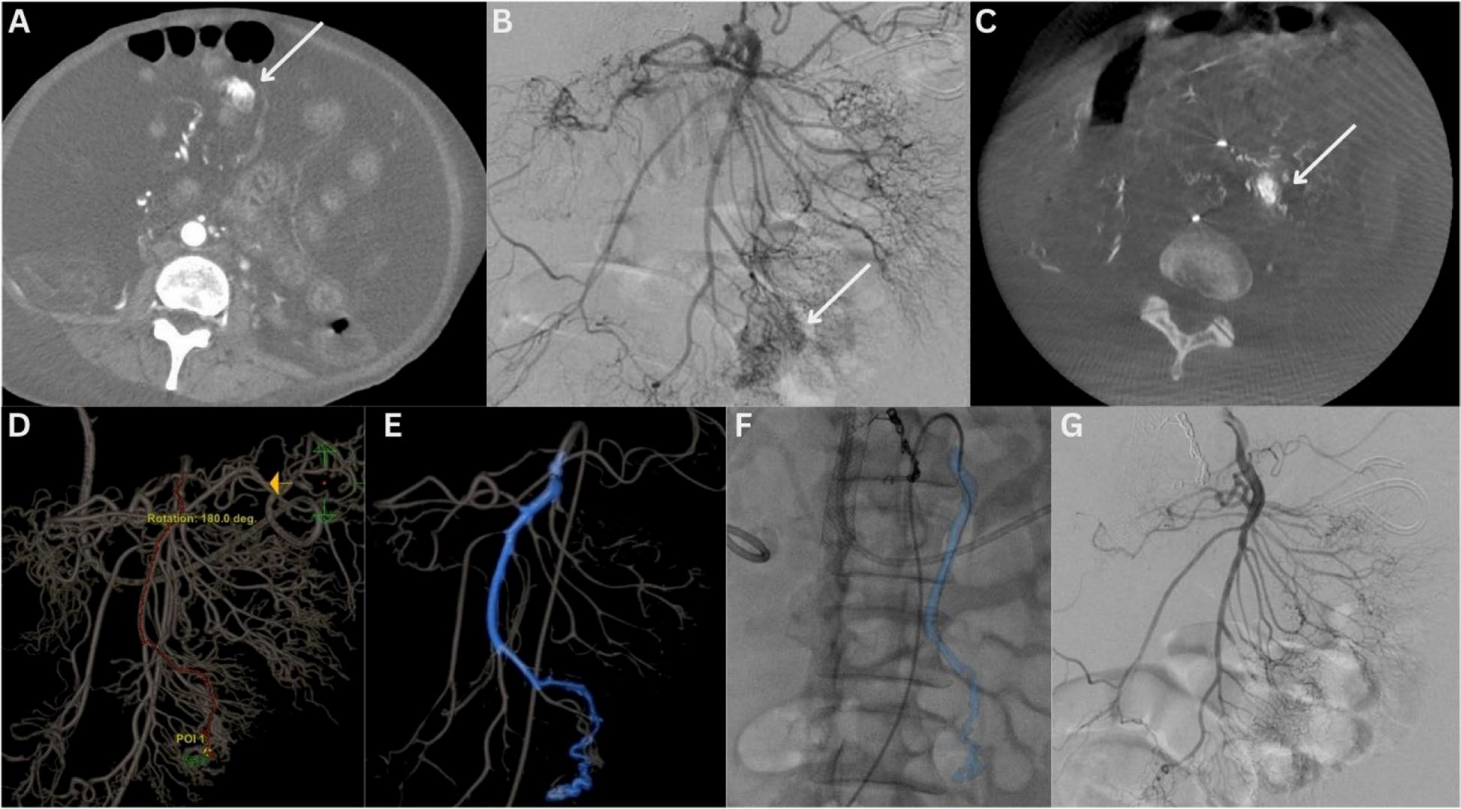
Case 1—Superior mesenteric artery embolization A 48-year-old woman presenting with lower gastrointestinal bleeding. A Focus of contrast extravasation (arrow) noted on the patient’s pre-procedural CT Angiogram. B No definitive focus of extravasation on a selective superior mesenteric artery (SMA) DSA. In retrospect an area of hypervascularity is noted in relation to an ileal branch (white arrow). C Given the patient’s large volume ascites and small bowel motion, the area of vascular abnormality (white arrow) now appeared to be positioned more centrally within the abdomen on the intra-procedural CBCT. D The arterial tree was automatically segmented and the point of interest was selected with automatic path calculation from the desired proximal position (Embo ASSIST with Virtual Injection, GE HealthCare, Chicago, IL, USA). E A colored three-dimensional roadmap is then generated from the processed data which can then be overlaid onto the live fluoroscopic image (F). G Post-glue embolization SMA DSA demonstrates successful post-embolization appearances
